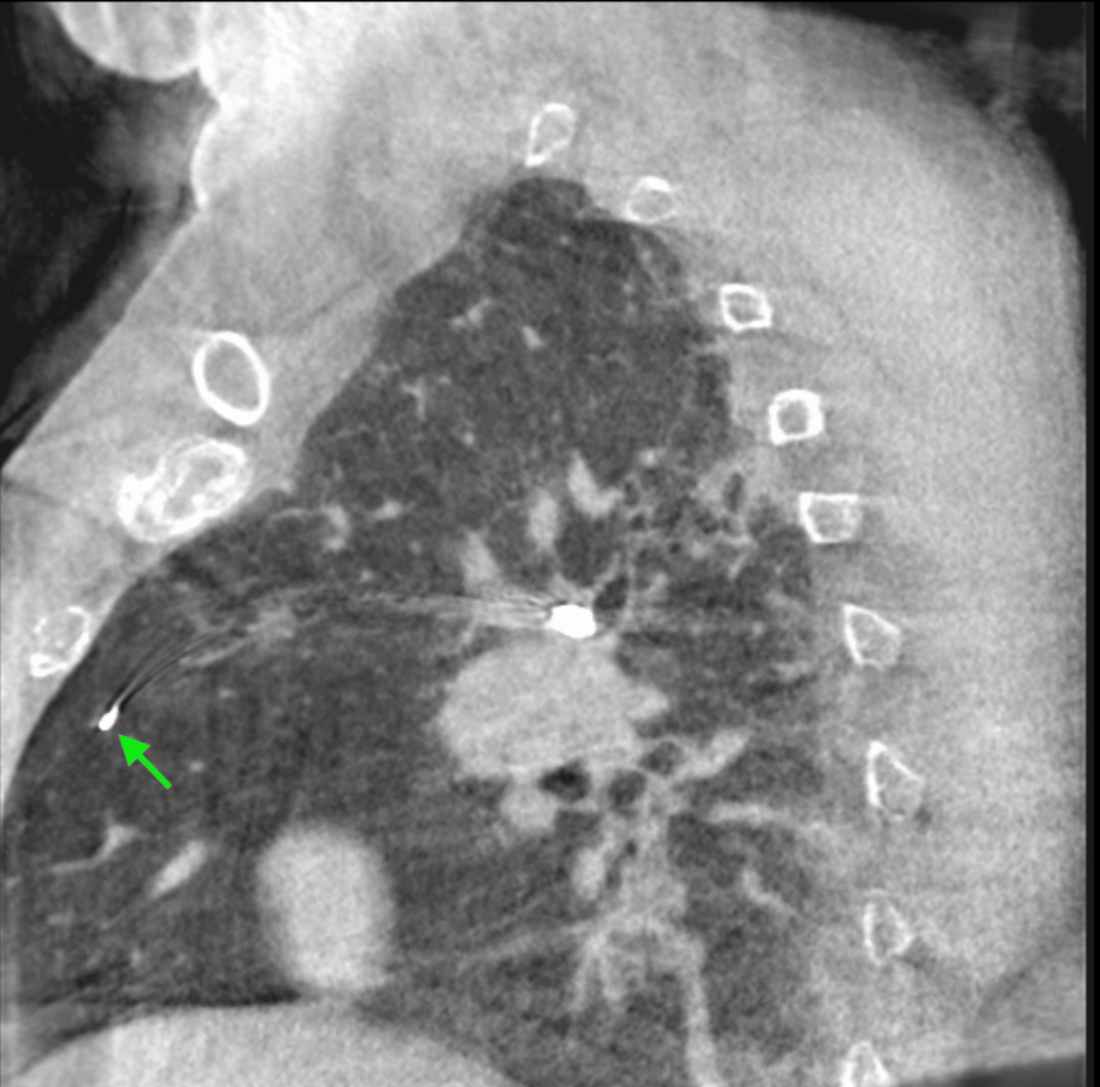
CBCT image of brush catheter adjacent to pleura (sagittal view). CBCT: cone-beam computed tomography
